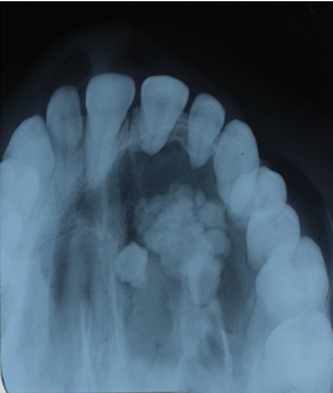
Occlusal radiograph showing radiopacities in left maxillary quadrant
